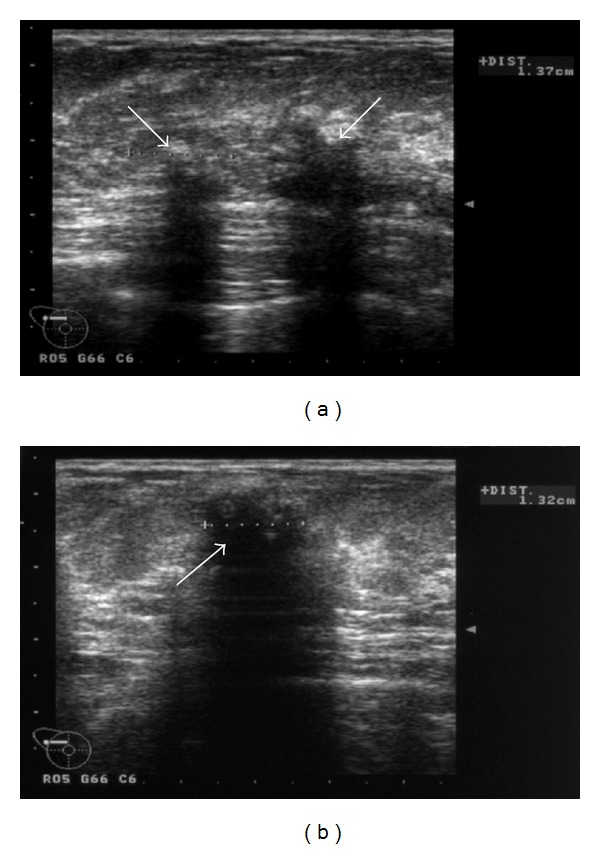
Ultrasound shows multiple, ill-defined hypoechoic areas with acoustic shadowing, indicating multiple coarse calcifications within the right breast (arrows).
